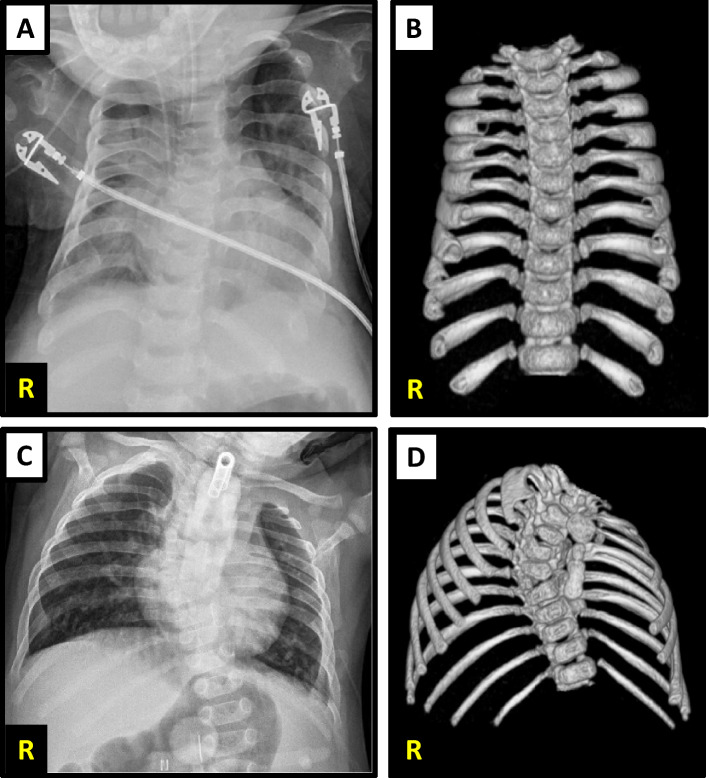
Patient 1 (A) Chest X-ray obtained at 2 years of age showing narrow thorax with short ribs and lung hypoplasia. (B) 3D reconstruction of the thorax from chest CT images obtained at 2 years of age. Patient 2 (C) Spine films obtained at 9 months of age showing thoracic scoliosis, thoracic spine vertebral segmentation defects, left rib fusion defects, and left lung compression. (D) 3D reconstruction of the thorax from chest CT images obtained at 1 year of age.
